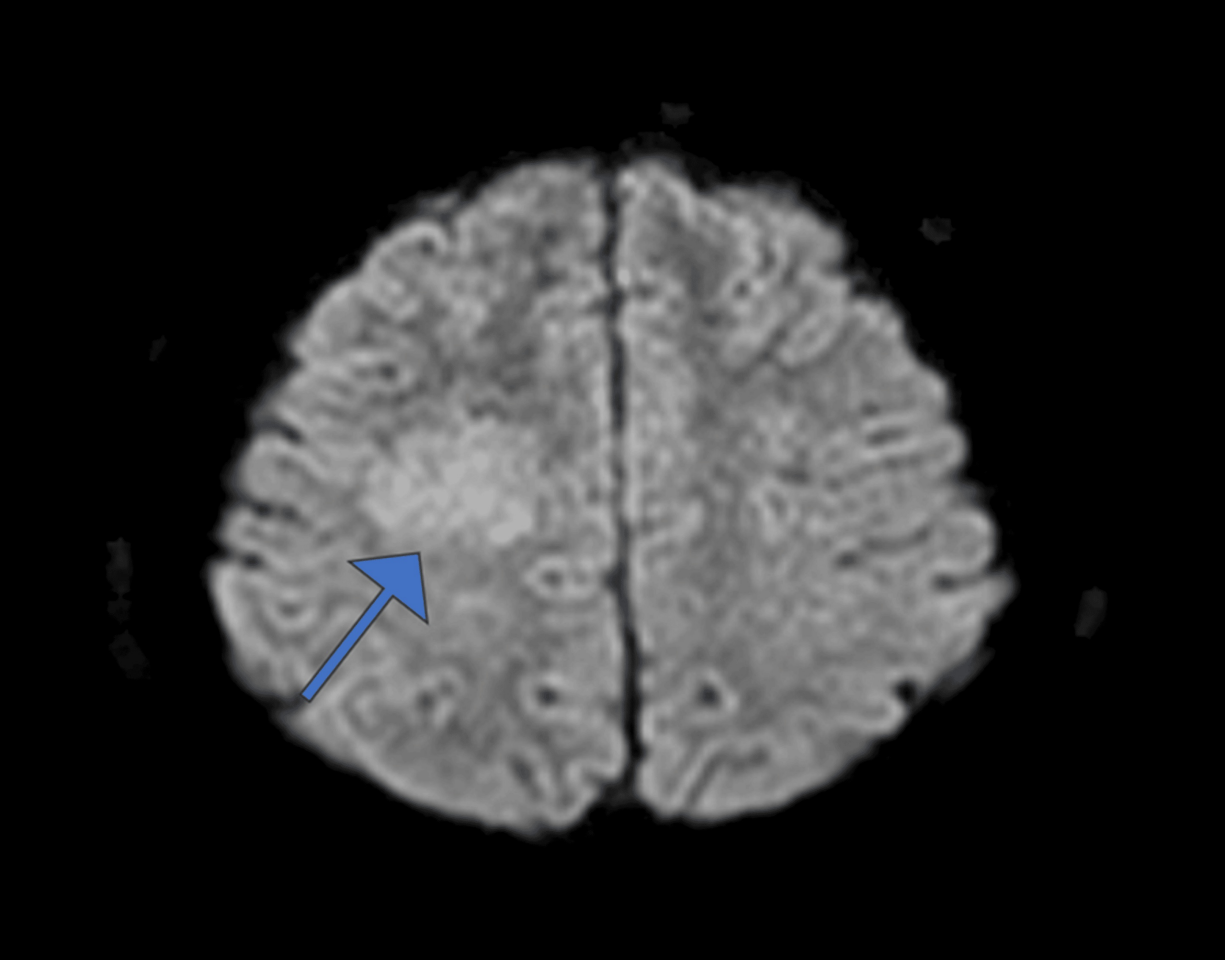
Axial diffusion-weighted MRI image showing no significant diffusion restriction at the lesion site (arrow).
